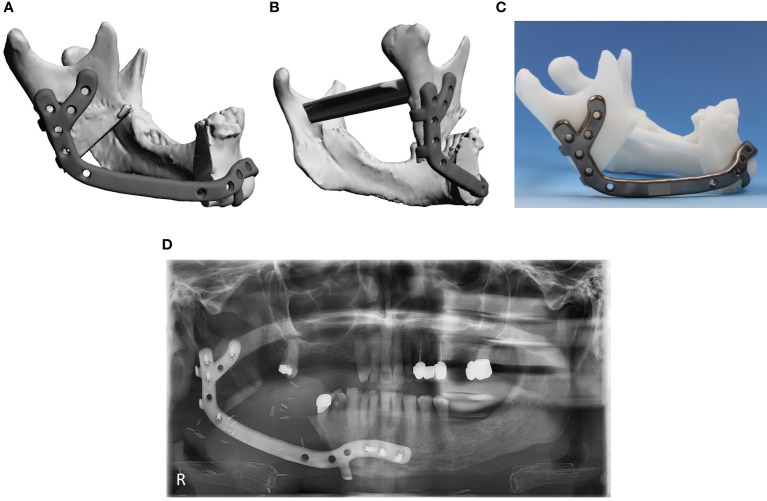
Complex patient-specific implant. (A) digital planning lateral view, (B) digital planning posterior-lateral view, (C) patient-specific implant on plastic model, (D) postoperative orthopantomogram.
